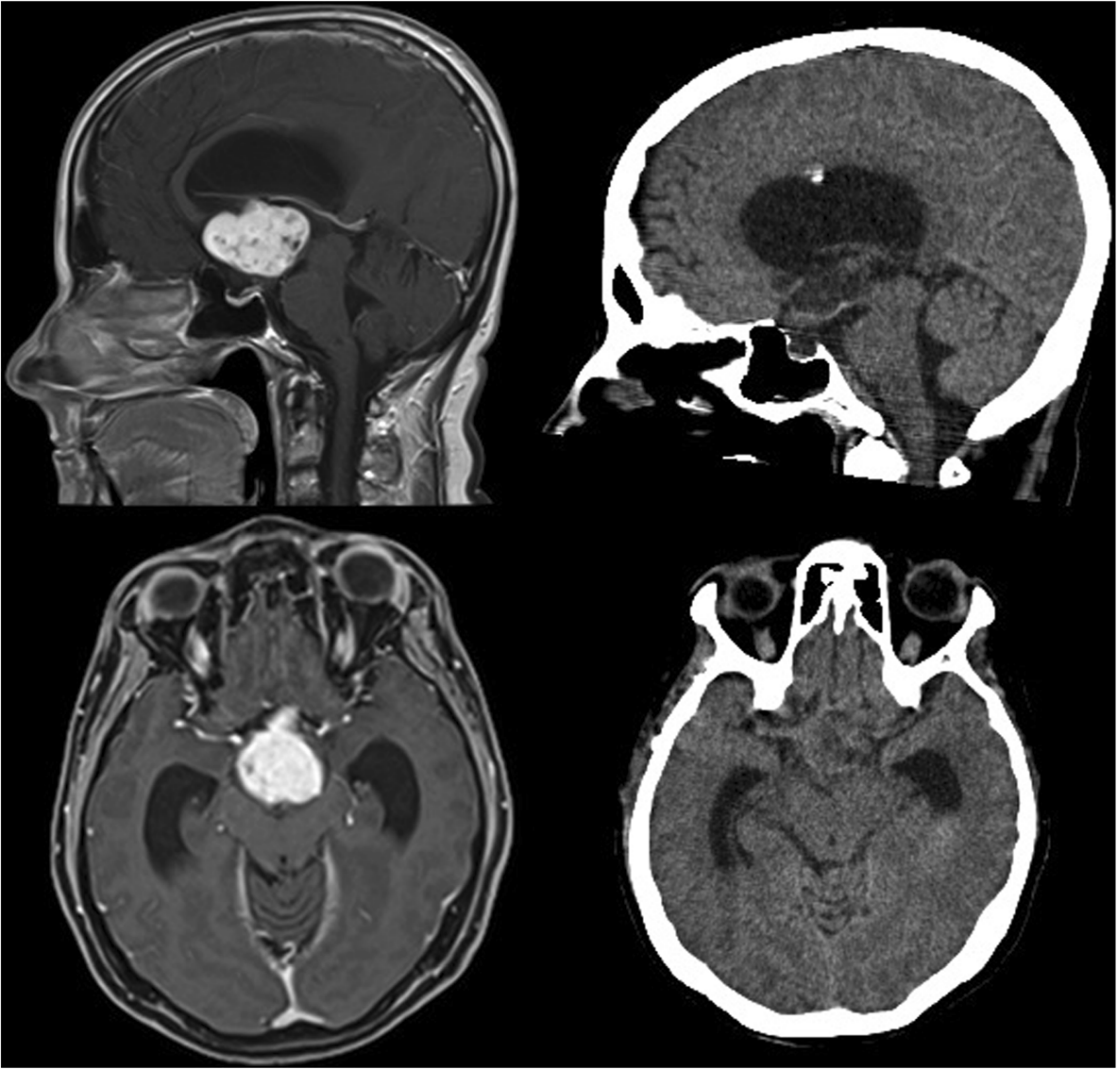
Radiological evaluation. Left: T1-weighted MRI scan showing a lesion in the medial mesencephalic region with heterogeneous contrast enhancement. Center: early postoperative CT scan showing gross total removal
